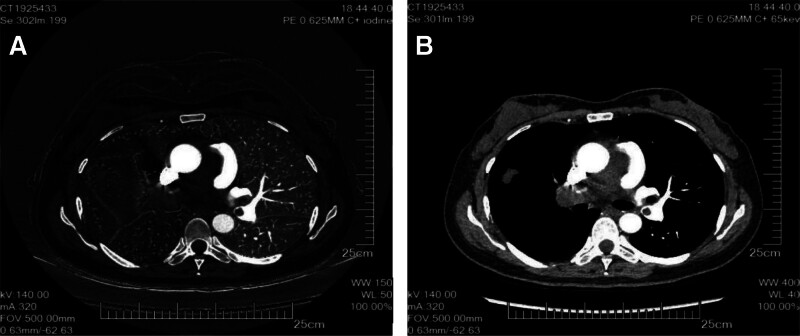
CTPA window and mediastinal window both show filling defects of the main pulmonary artery trunk and right and left pulmonary artery trunks A: pulmonary window; B: mediastinal window. CTPA = computed tomography pulmonary angiography.
